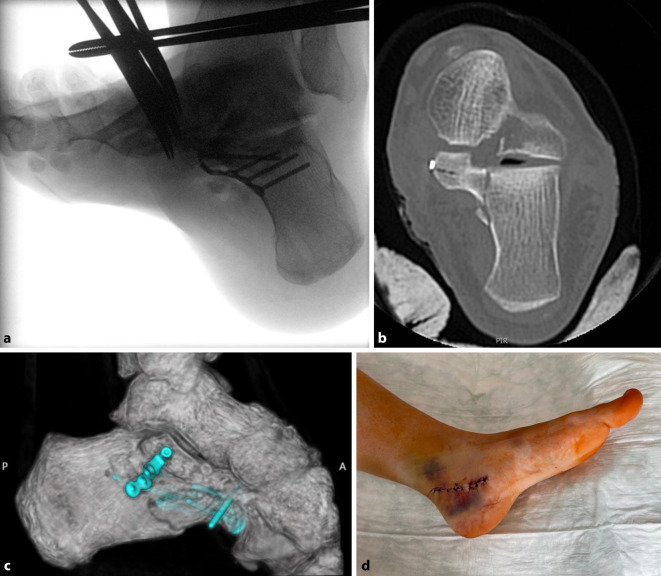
Schrauben- und Abstützplatten-Osteosynthese bei dislozierter Sustentaculum-Fraktur über einen McReynolds-Zugang. a Intraoperativer Broden-View, b paraaxialer CT-Schnitt auf Höhe des Sustentaculum tali, c 3D-CT-Rekonstruktion und d klinisches Bild des Zugangs nach Reposition und Osteosynthese
